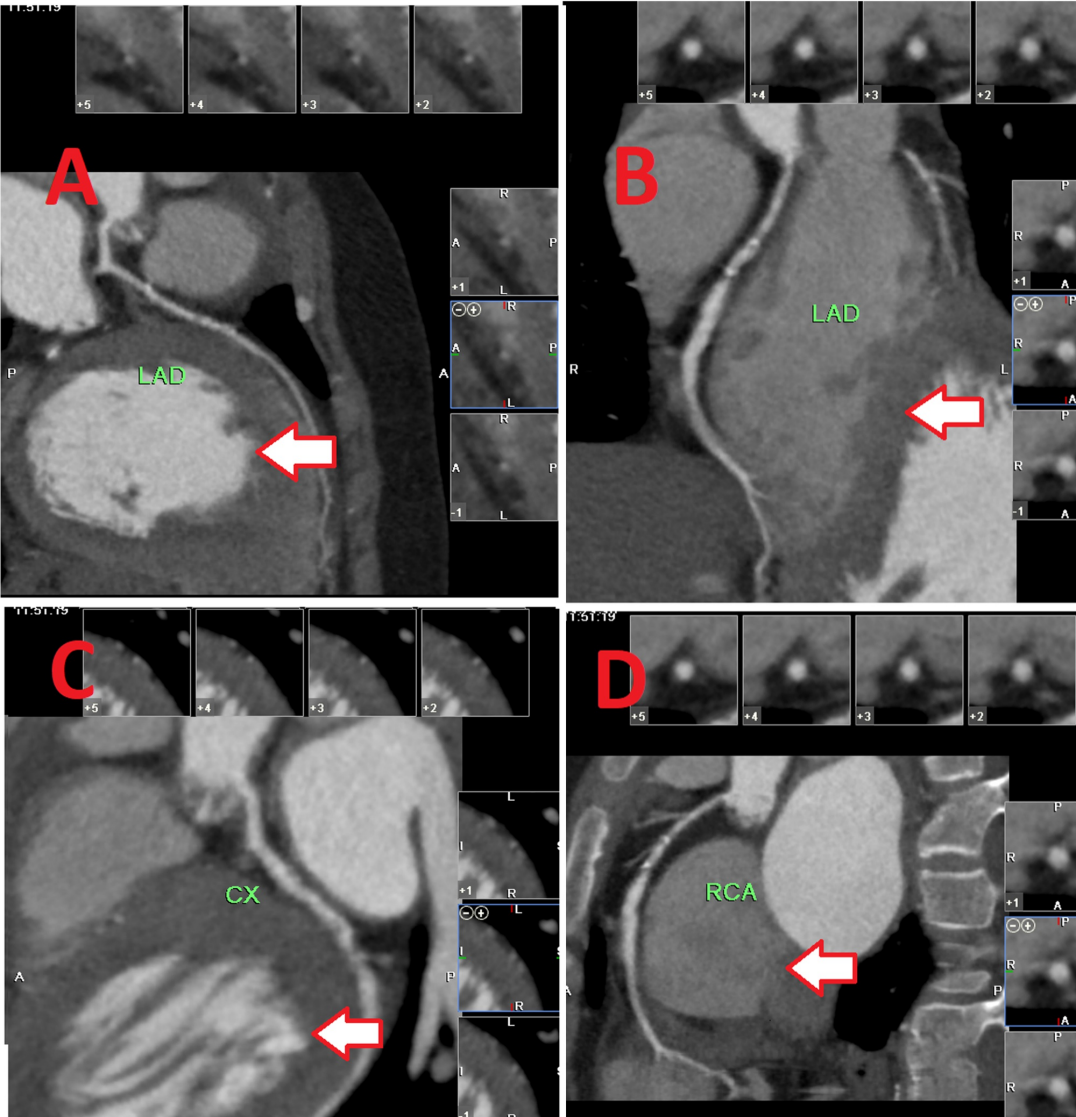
CT coronary angiogram A) left anterior descending artery (horizontal section) with moderately severe mid disease; B) left anterior descending artery (vertical section) with moderately severe mid disease; C) left circumflex artery with mid severe disease; D) right coronary artery with mild ostio-proximal disease CT: computed tomography
